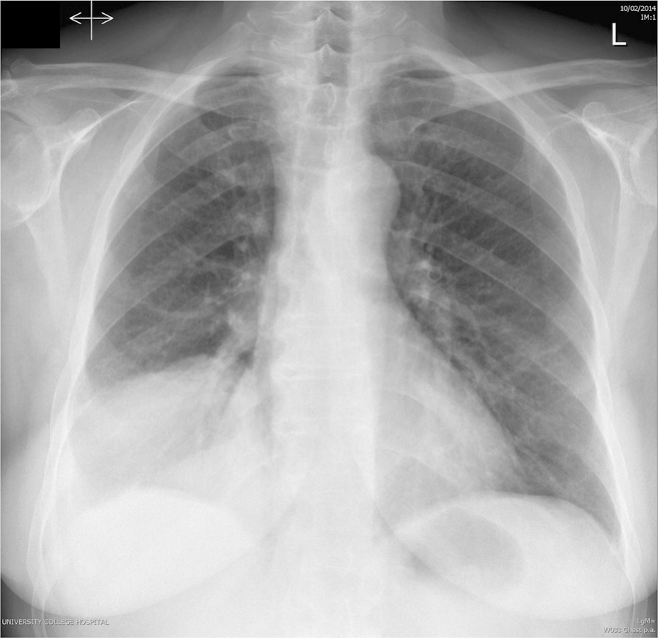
Plain chest radiograph showing a hydropneumothorax on the right side with the pneumothorax component measuring 16 mm. A high right hemidiaphragm is also evident
